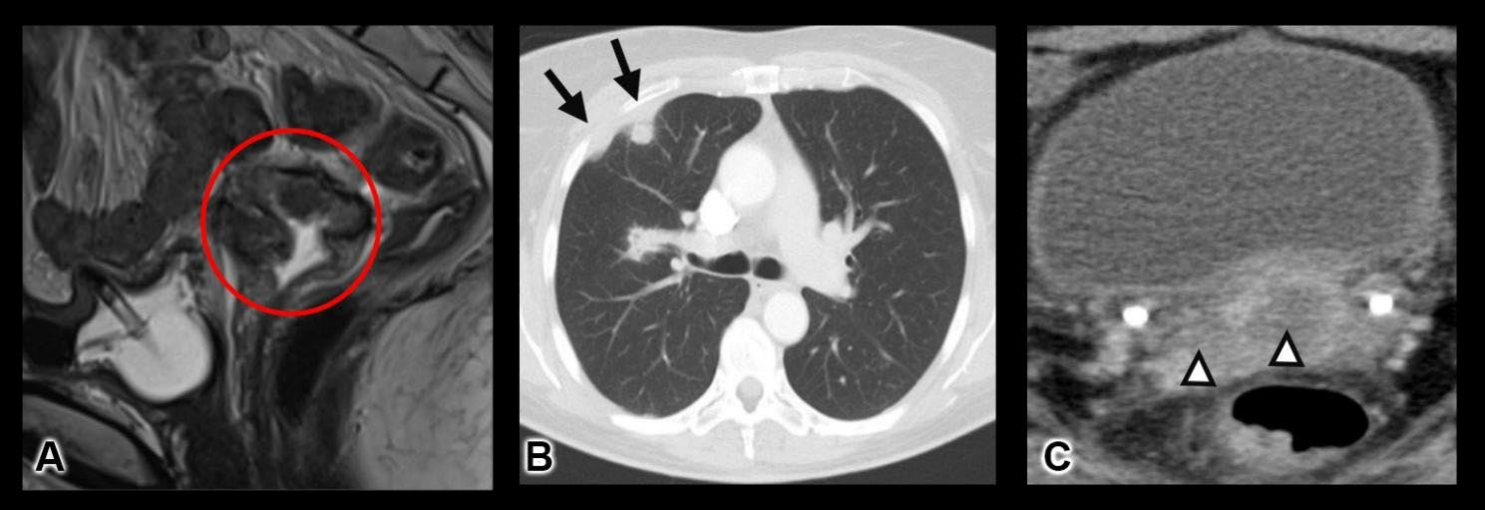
A) Sagittal T2-weighted MRI after radiochemotherapy shows satisfactory decrease in tumor size. B) Axial contrast-enhanced CT scan shows a solid nodule with spiculation in the posterior segment of the right upper lobe. Moderate pleural thickening with a pleural-based nodule in the anterior segment of the same lobe. C) Axial contrast-enhanced CT scan shows the cervical tumor with areas of encapsulated necrosis
